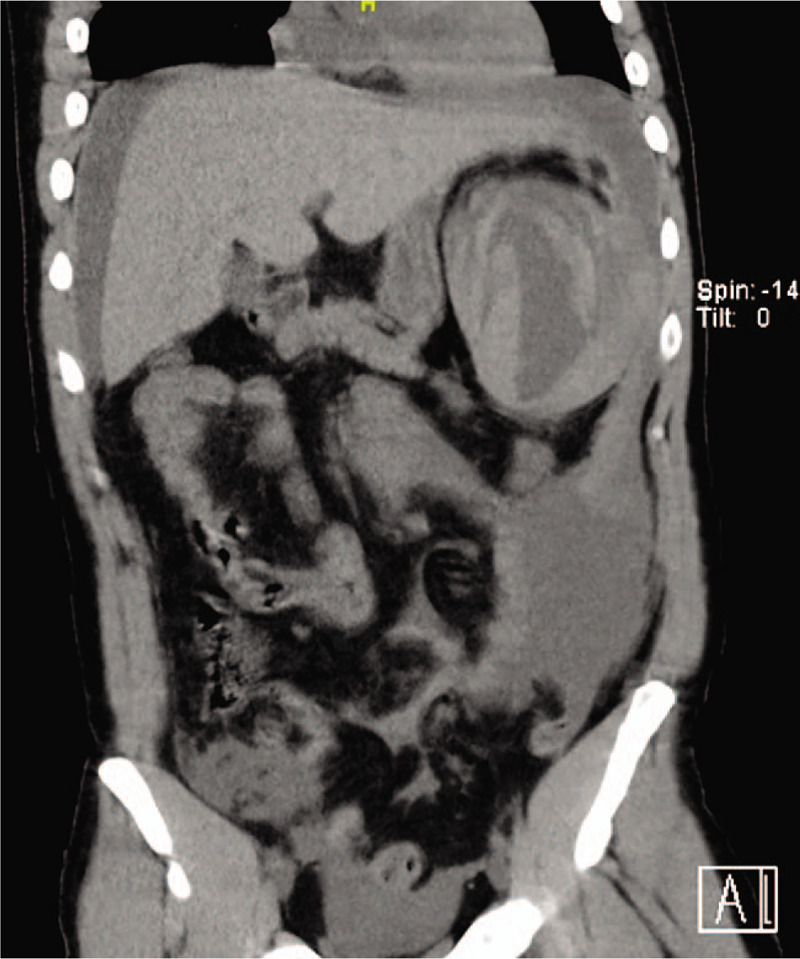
Computed tomography scan showed shows that the spleen was is enlarged and ruptured. The arrow denotes that there were are multi-focal hemorrhages in the capsule and parenchyma.
